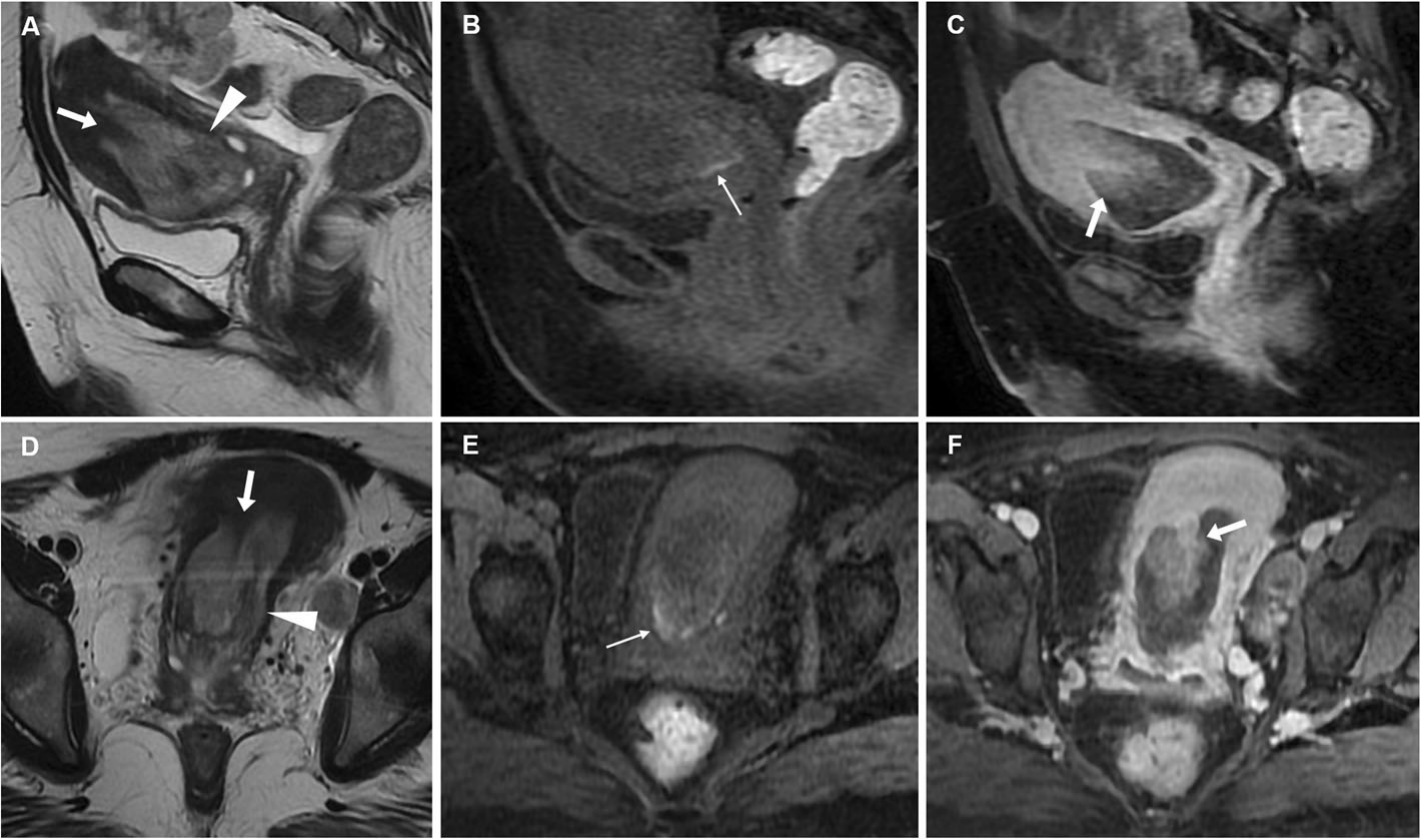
Endometrial polyp in a 44-year-old woman with acute uterine bleeding and associated bilateral ovarian neoplasm. Sagittal (a) and oblique-coronal (d) T2-weighted images show a huge polypoid mass (arrowheads) arising from the uterine fundus that fills the endometrial cavity and protrudes into the cervical canal. Note the hypointense stromal axis of the polyp (arrows). Corresponding sagittal (b) and oblique-coronal (e) precontrast fat-suppressed T1-weighted images show hyperintense foci on the polyp edge (thin arrows), representing haemorrhage. On gadolinium-enhanced sagittal (c) and oblique-coronal (f) fat-suppressed T1-weighted images, the implant base (stromal axis) of the polyp shows intense enhancement (arrows). The patient underwent bilateral uterine artery embolisation in order to control bleeding
